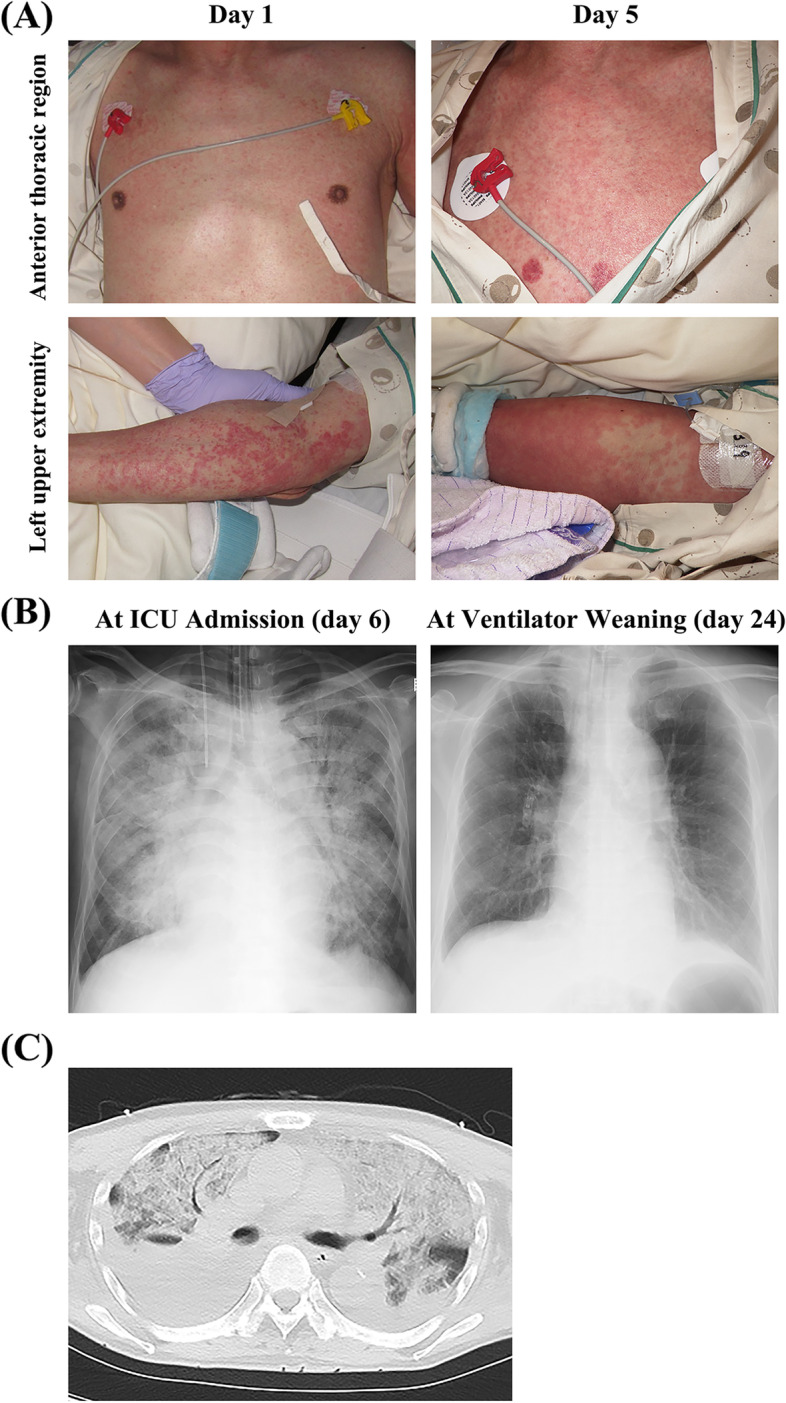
Skin findings on day 1 and day 5 and chest radiograph and CT. Lesions in A the anterior thoracic region and upper left extremity. Miliary-sized erythema was observed on the extremities and trunk on day 1 (A). On day 5, there was expansion of the skin rash. B Chest radiography upon ICU admission (day 6, left panel) and ventilator weaning (day 24, right panel). The decreased permeability of the entire lung field, which could have been caused by the infiltrated fluid observed at ICU admission, improved at the time of weaning from ventilation. C Chest computed tomography (CT) at ICU admission. Frosted shadows in all lung fields and a large pleural effusion were observed
